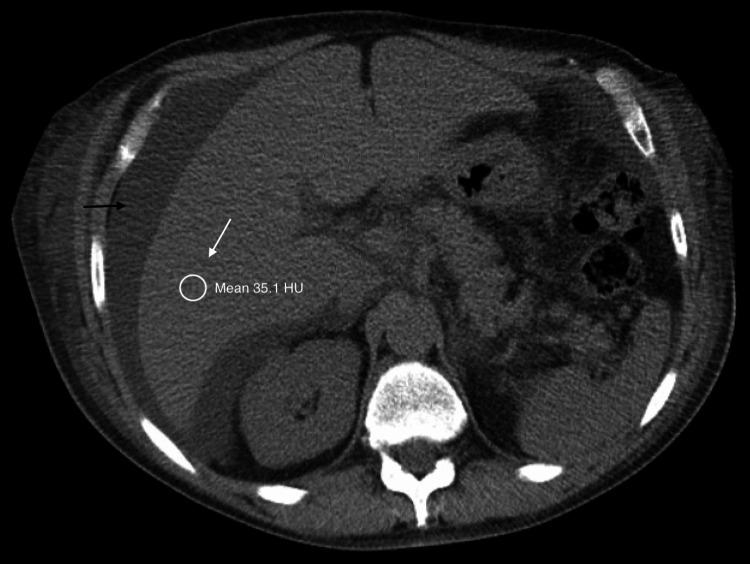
Non-contrast CT abdomen showing ascites (black arrow) and diffusely decreased hepatic attenuation (white arrow). Abbreviations:  HU, Hounsfield unit.
